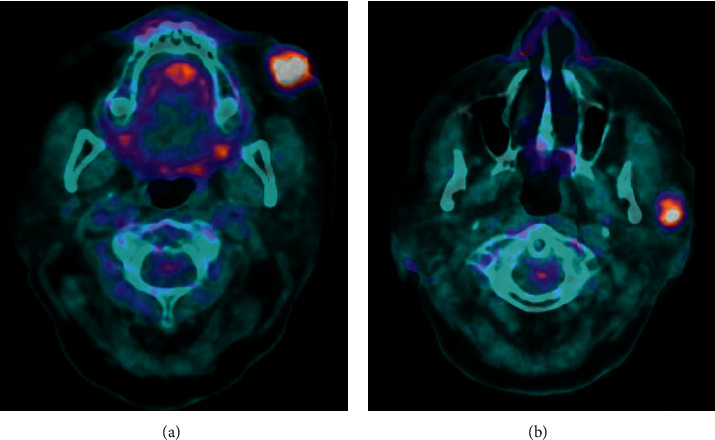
Staging PET/CT scan. (a) Primary site of disease to the left cheek. (b) Regional metastasis to the left parotid.
